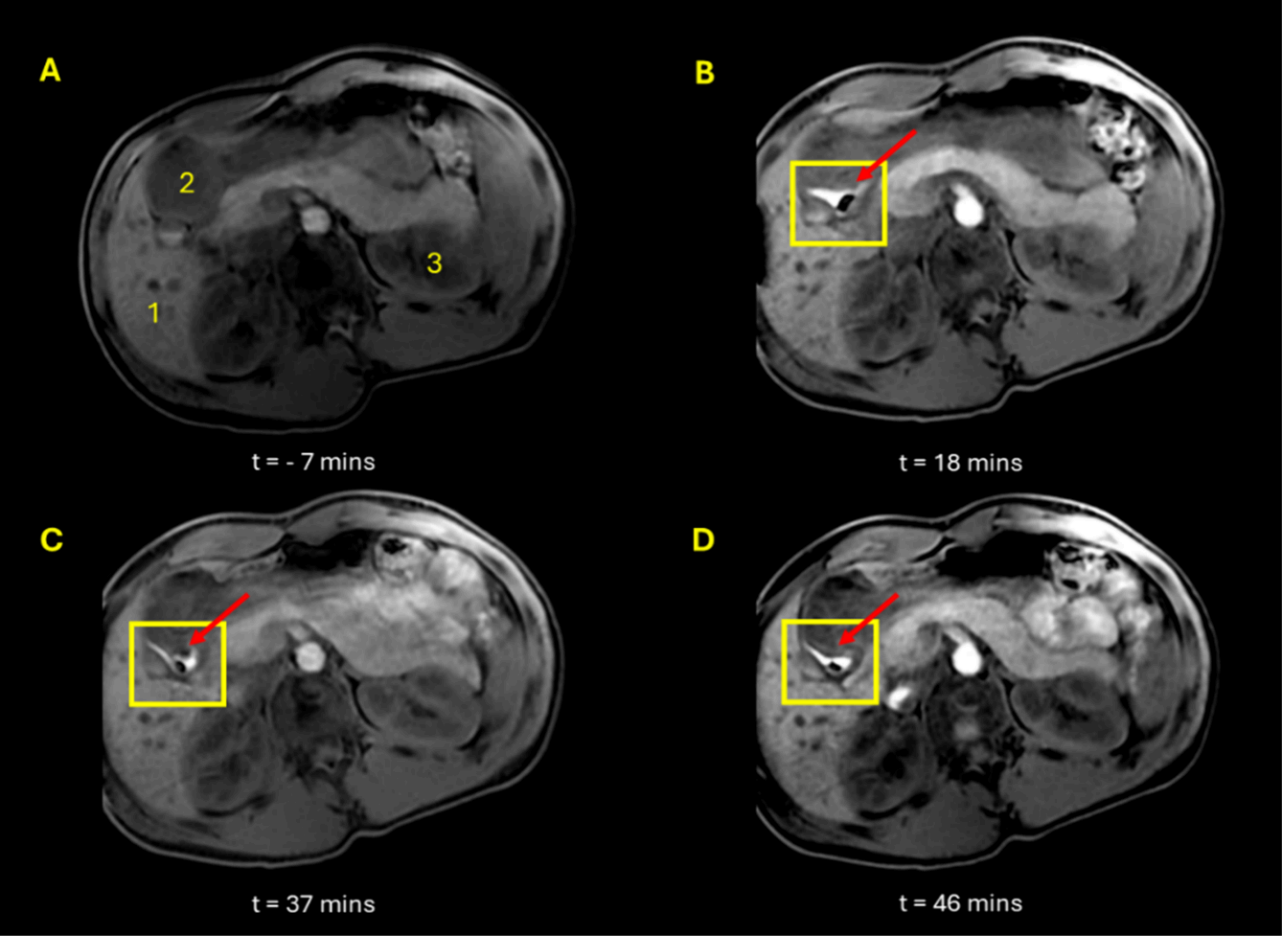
Axial, 3D LAVA MRI images
